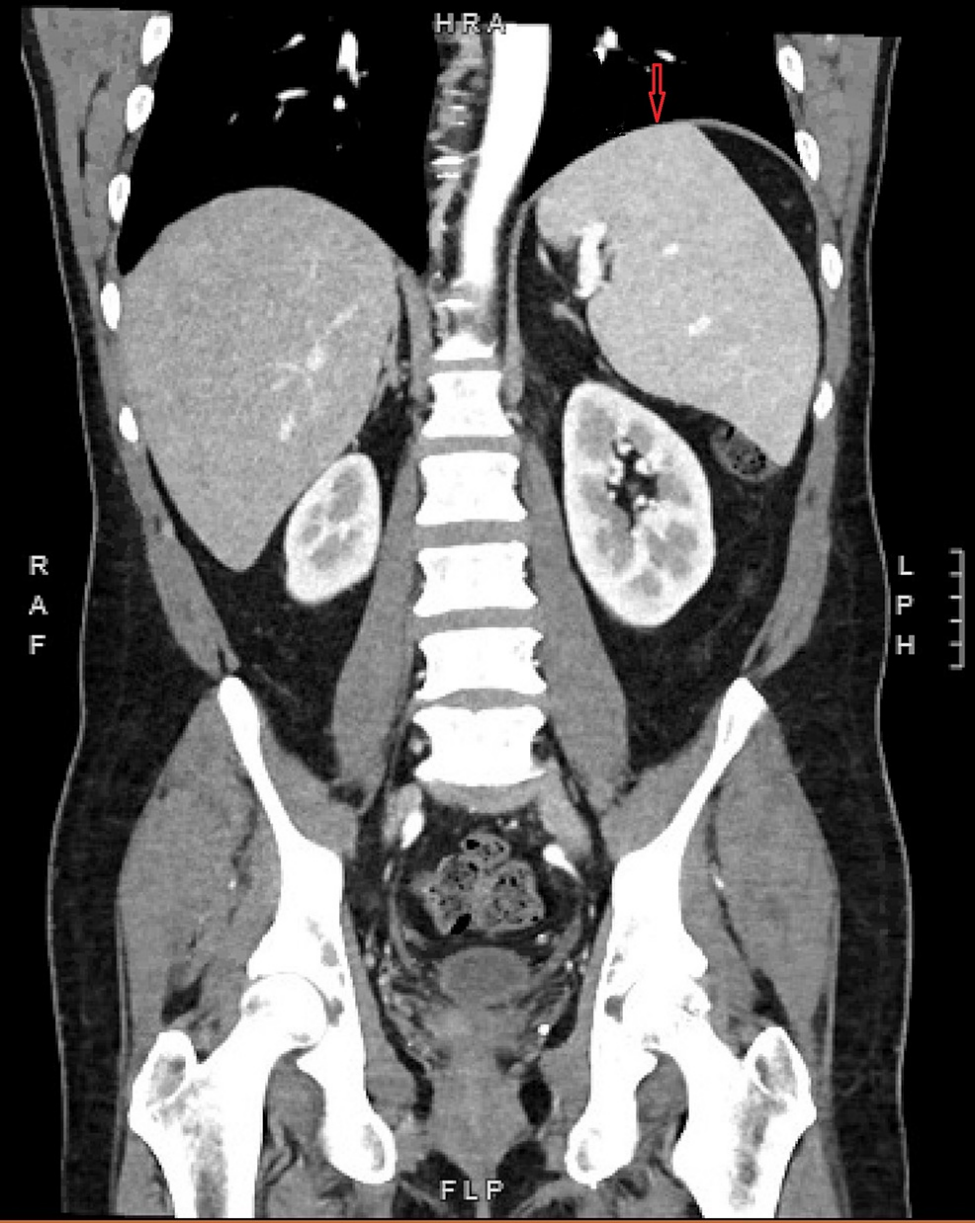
Coronal plane CT of the abdomen and pelvis demonstrating splenomegaly (red arrow) measuring 15.2 cm craniocaudally
